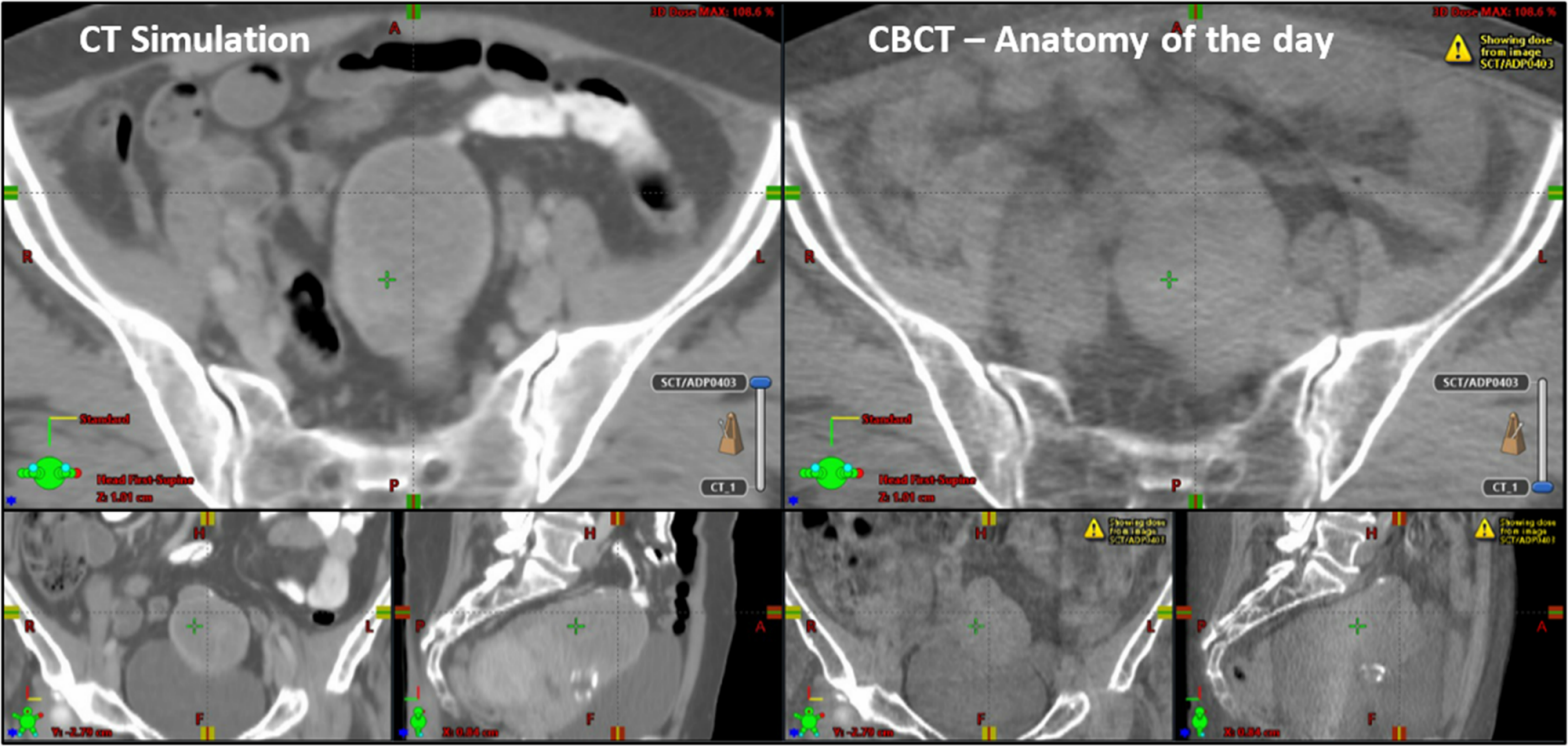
CT scan of patient 8 (left) and CBCT (right) 48 days later showing how some patients do not benefit extensively from adaptation due to their anatomy maintaining both shape and location through treatment. CBCT, cone‐beam computed tomography
